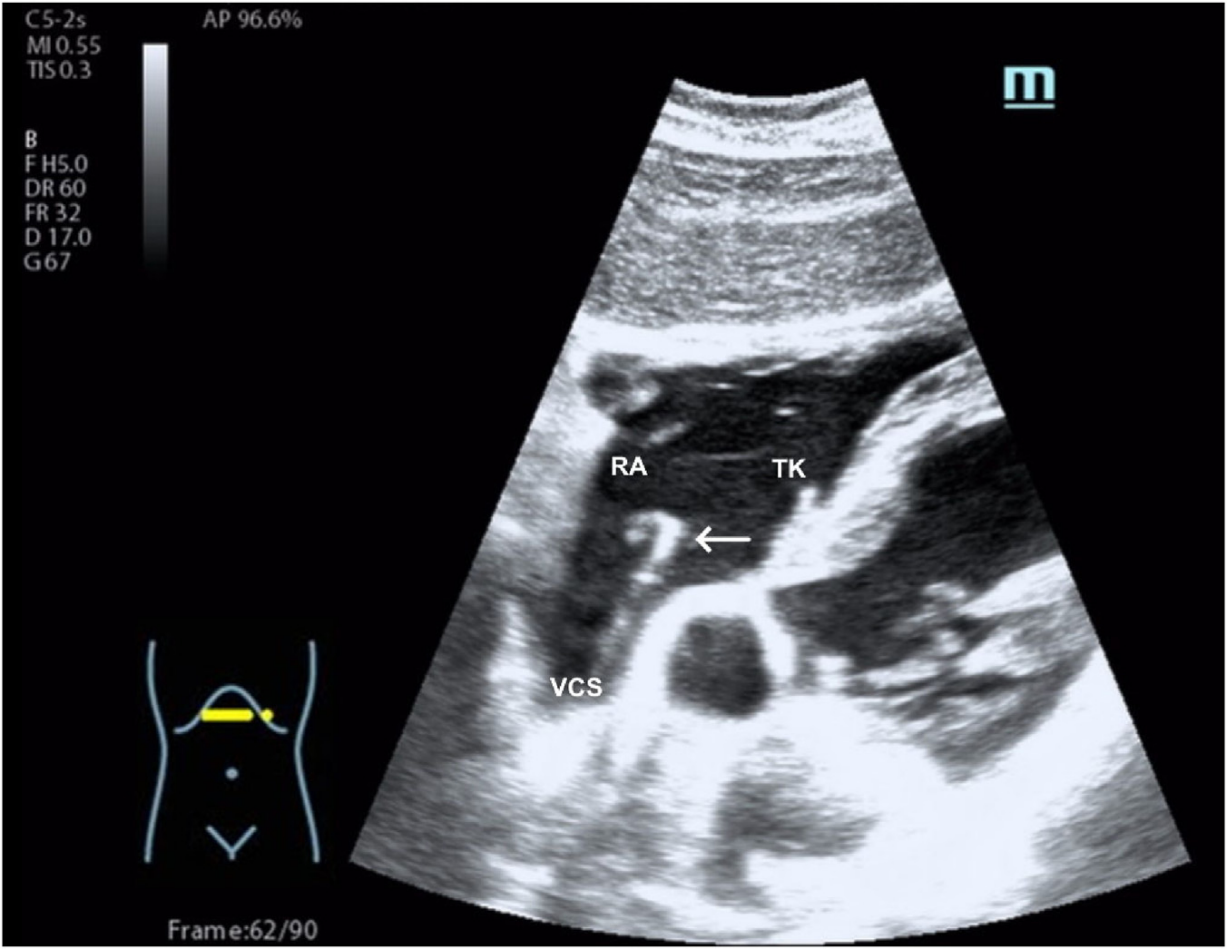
Ultrasonographic visualization of the right atrium for catheter positioning. Ultrasonographic visualization of the right atrium for catheter positioning. Catheter and guidewire are aligned with the J protruding from the catheter tip for a better contrasting of the catheter tip in the middle right atrium. RA, right atrium; TK, tricuspid arteriovenous valve; VCS, superior vena cava; Arrow, catheter tip with J-wire.
